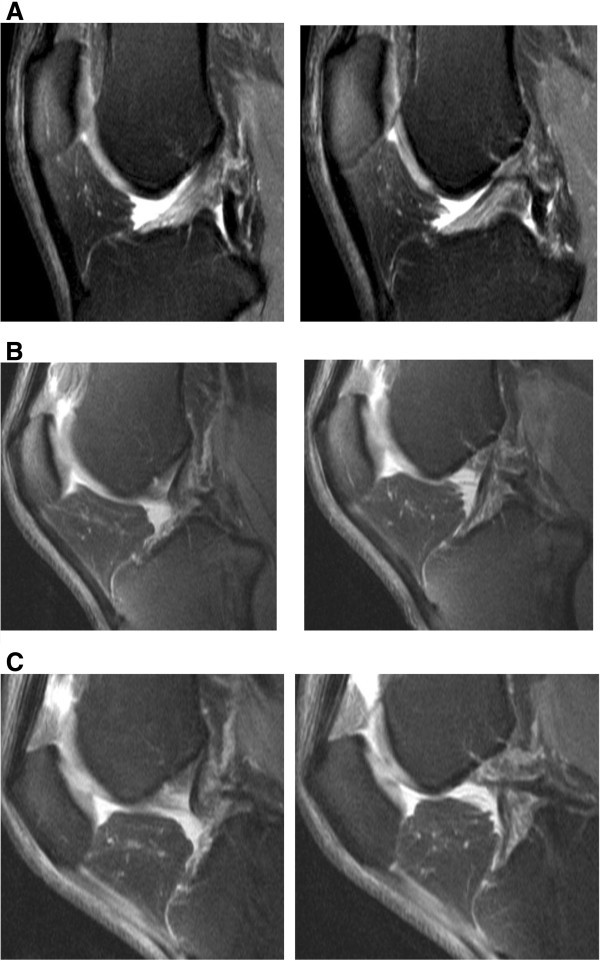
Normal ACL images obtained at knee extension (A), 30° of knee flexion (B) and 55° of knee flexion (C) on T2-weighted TSE FS sagittal image (2900/90). At extension (A) the ACL fibers are seen as linear, striate fibers extending from the roff of the femoral notch to the tibial surface. At 30° and 55° of flexion (B, C) a lengthening with astraing-like appearance of the ACL is seen. The posterior ACL, changes its appearance from an angular-konvex contour at extension (A), to a more straight like appearance with increased flexion angles (B, C).
